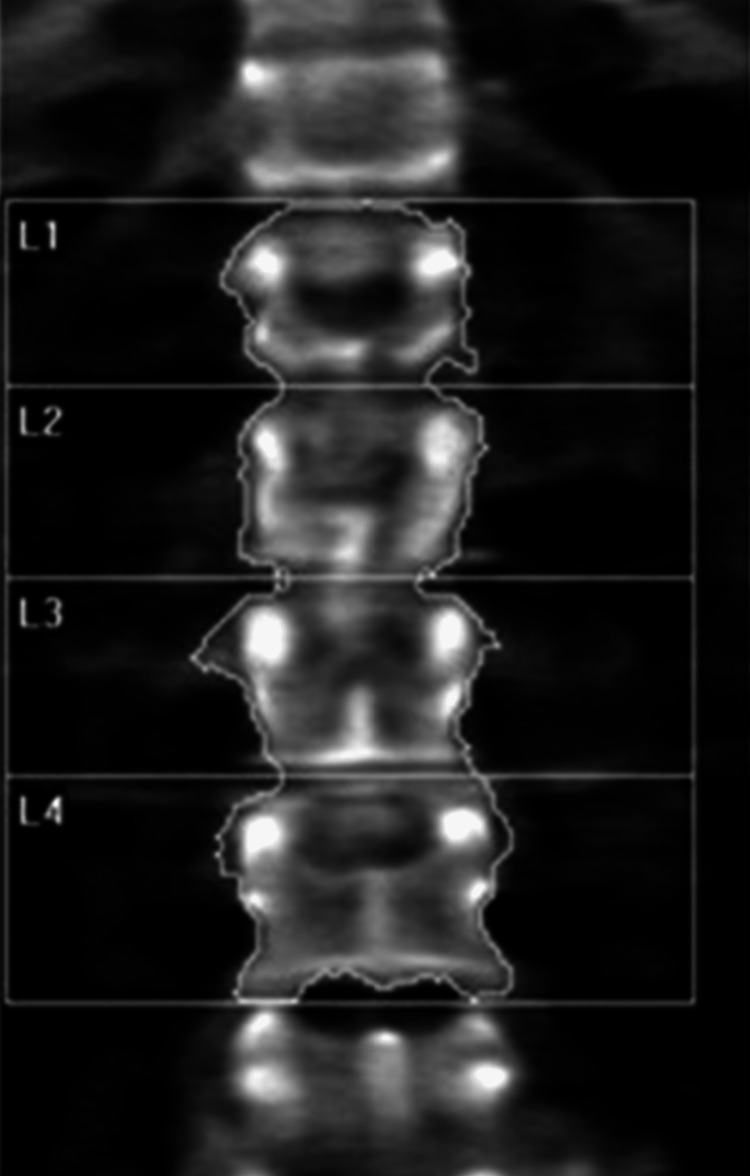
Dual-energy X-ray absorptiometry (DEXA) scan of the lumbar spine showing osteoporosis
